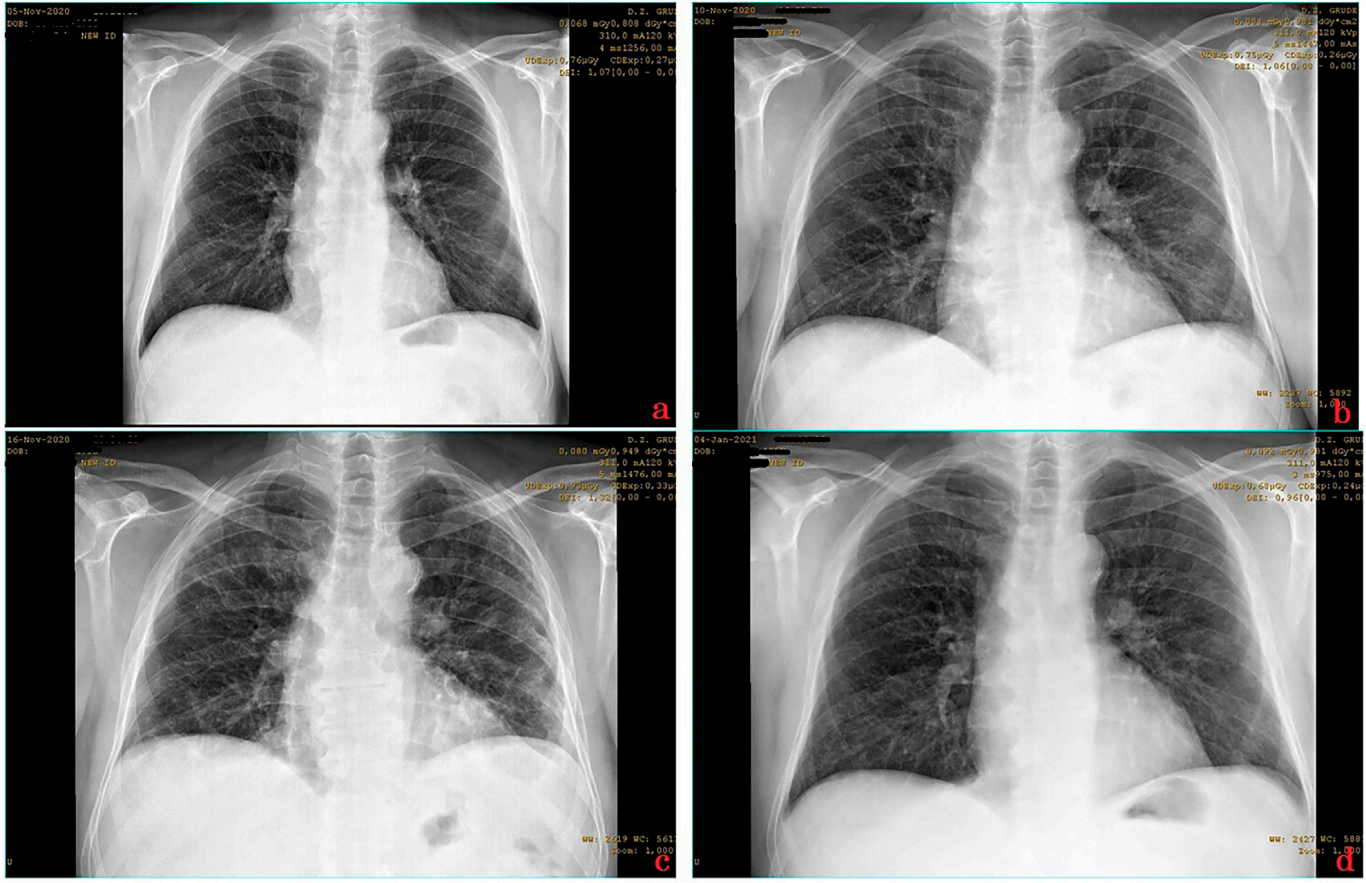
Series of chest X-ray images of COVID-19 patient from case 3. The images are chronologically* arranged in a–z order (the letter mark is indicated in the lower right corner of each image)^§^. *The dates of records are visible in the top right corner of each image. ^§^Identifiable patient’s data are covered.
